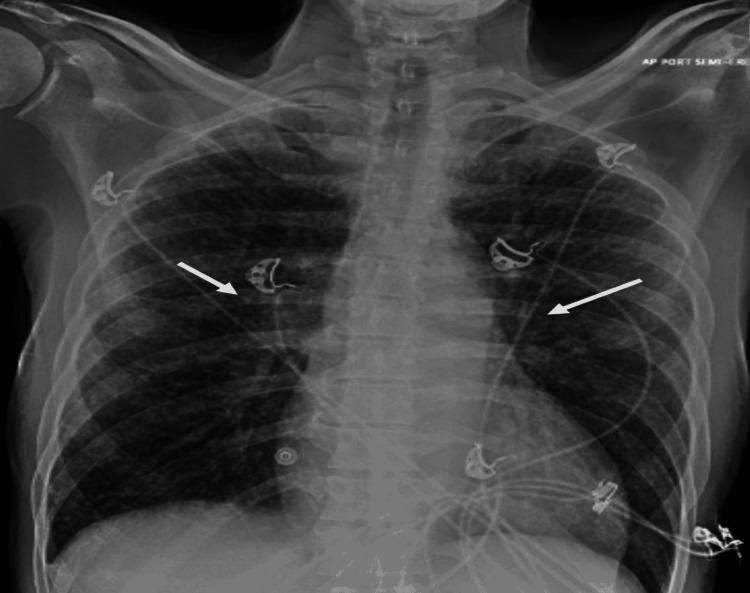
Chest X-ray (anteroposterior view) on presentation. White arrows show bilateral widespread fine reticulonodular opacities.
